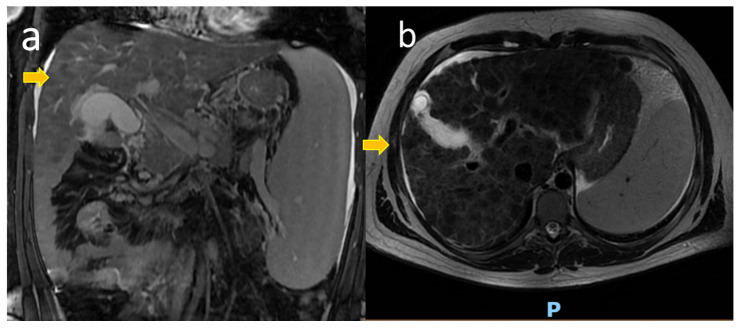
Upper abdominal Magnetic Resonance Imaging (MRI) of Patient n°1: (a) Coronal T2 weighted section; (b) Axial T2 weighted section; diffusely hyperintense hepatic nodules are evident, as indicated by the arrows. LEGEND: P = posterior.
